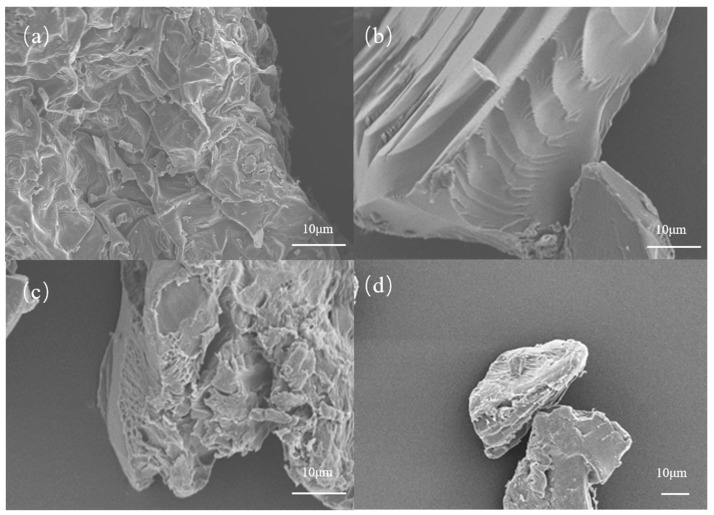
Surface characteristics of (a) CT, (b) CK, and (c,d) CT-gel.
